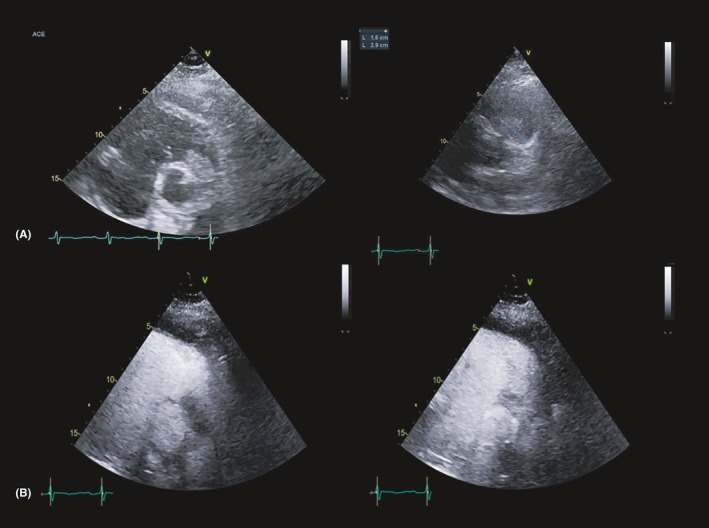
A, transthoracic echocardiography, parasternal short‐axis view. Hypoechoic mass at the level of right ventricular outflow tract is evident. B, contrast‐enhanced transthoracic echocardiography. Left: early acquisition after contrast administration, hypoechoic aspect of the mass. Right: late acquisition with significant enhancement of the mass
